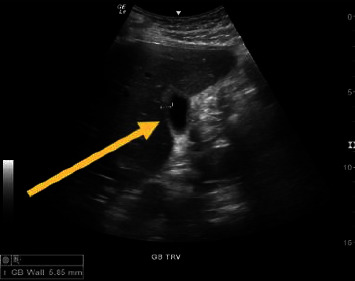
Right upper quadrant abdominal ultrasound on admission showing gallbladder wall thickening to 5.85 mm (indicated by yellow arrow; normal gallbladder wall thickness is less than 3 mm) and no gallbladder stones.
